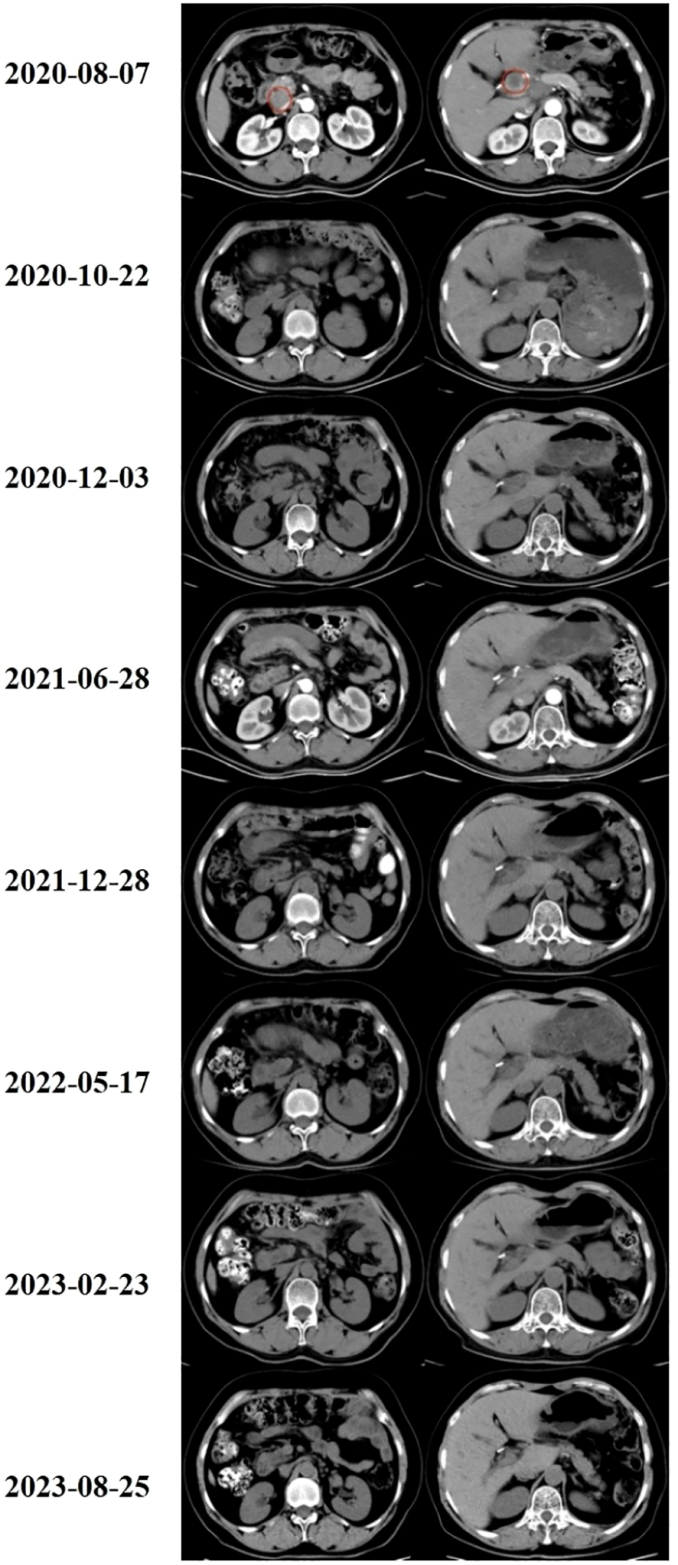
Abdominal CT scanning of the patient. (2020-08-07)The metastatic lymph nodes in the right posterior part of the hepatic artery were shown by CT before treatment. (2020-10-22 to 2023-08-25)After short-course hypofractionated radiation therapy combined with PD-1 inhibitors, CT revealed the disappearance of metastatic lymph nodes in the abdominal cavity. The therapeutic effect achieved a complete response.
